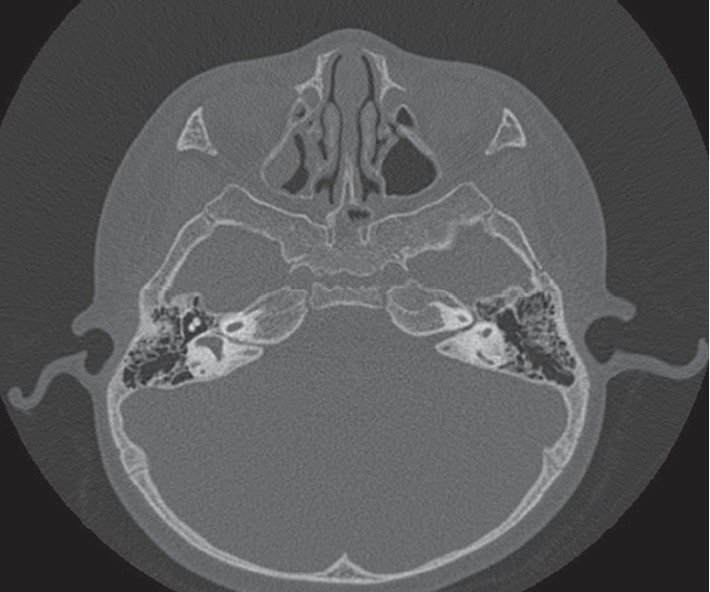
CT scan of the temporal bone with narrowed internal auditory canal (IAC).
